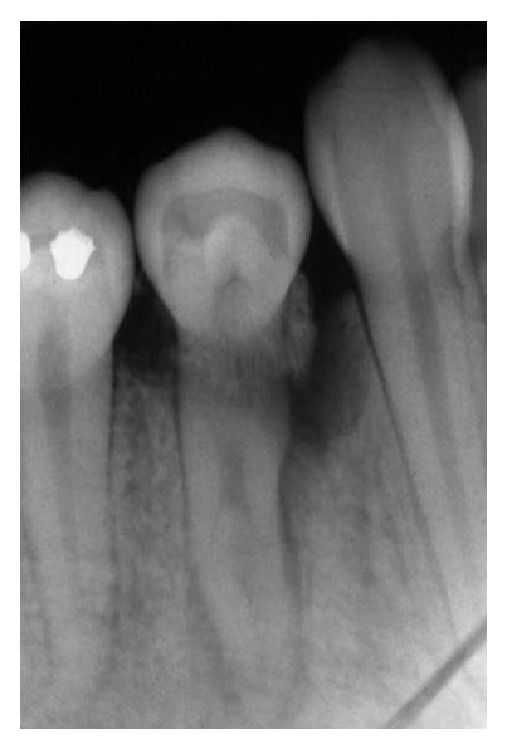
Radiograph of tooth 44. The presence of a radiopaque structure can be observed around the cervical region.
